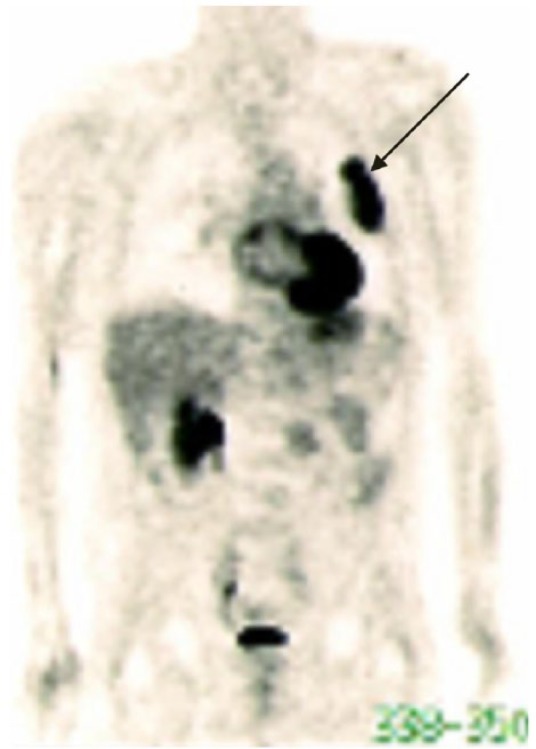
August 2010, a PET/CT scan showed a large pleural based mass in his left
